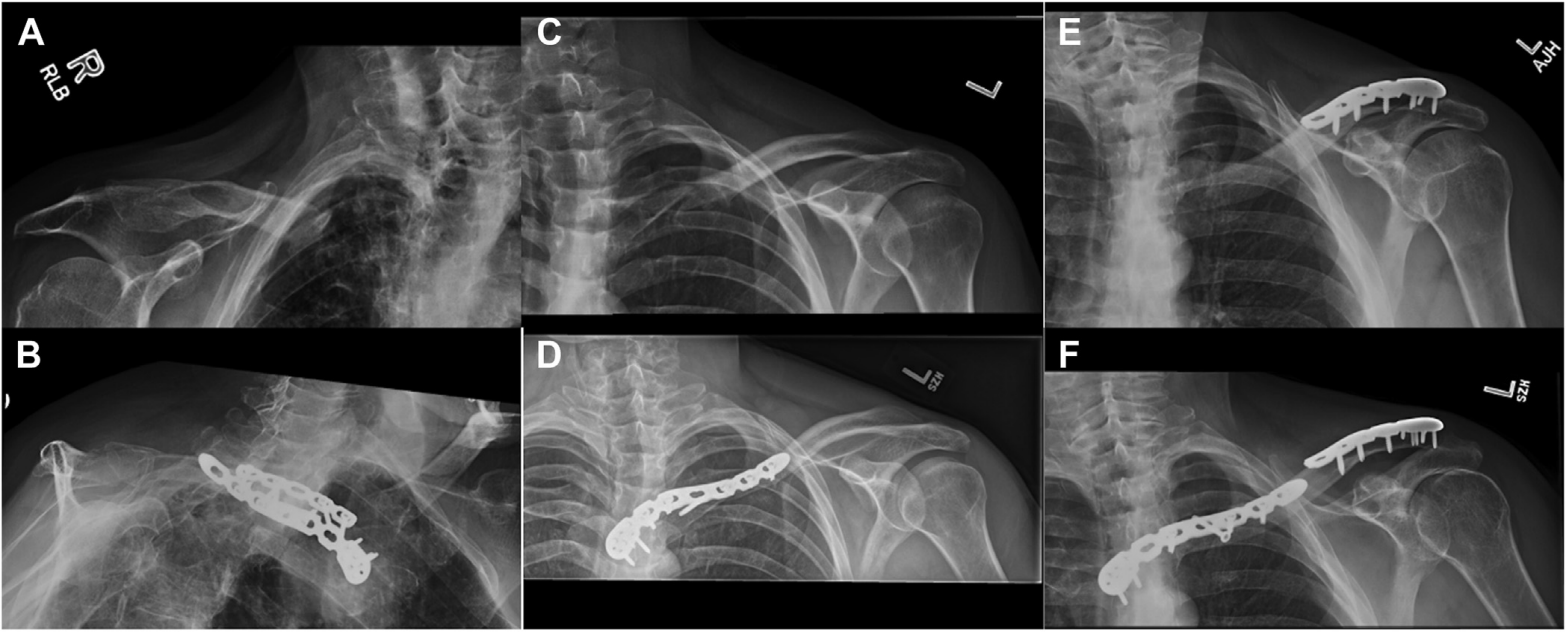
Preoperative and postoperative radiographs are shown for all three patients included in this report. Patient 1 is shown in (A) (preoperative) and (B) (postoperative). Patient 2 is shown in (C) (preoperative) and (D) (postoperative). Patient 3 is shown in (E) (preoperative) and (F) (postoperative).
